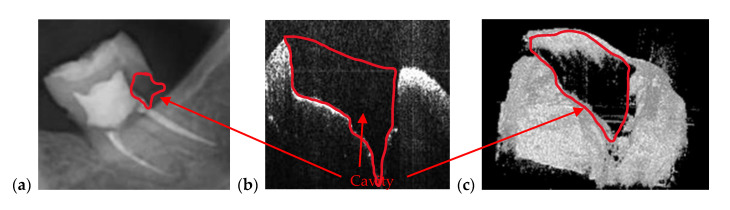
Cavity of a second molar from the third quadrant assessed on (a) a section cropped from a panoramic radiography. OCT investigation on the tooth extracted for medical purposes after performing the radiography: (b) B-scan and (c) 3D reconstruction. Patient M.N., female, age 29 years, diagnosed with a cavity on the smooth surface of the tooth and one of the tooth’s root. The latter is so large because there are two cavities connected with each other: The first one is a recurrent cavity that appeared under the filling because of an endodontic treatment; the second one appeared because of the receding gingiva and mandibular bone, which has left the tooth exposed.
